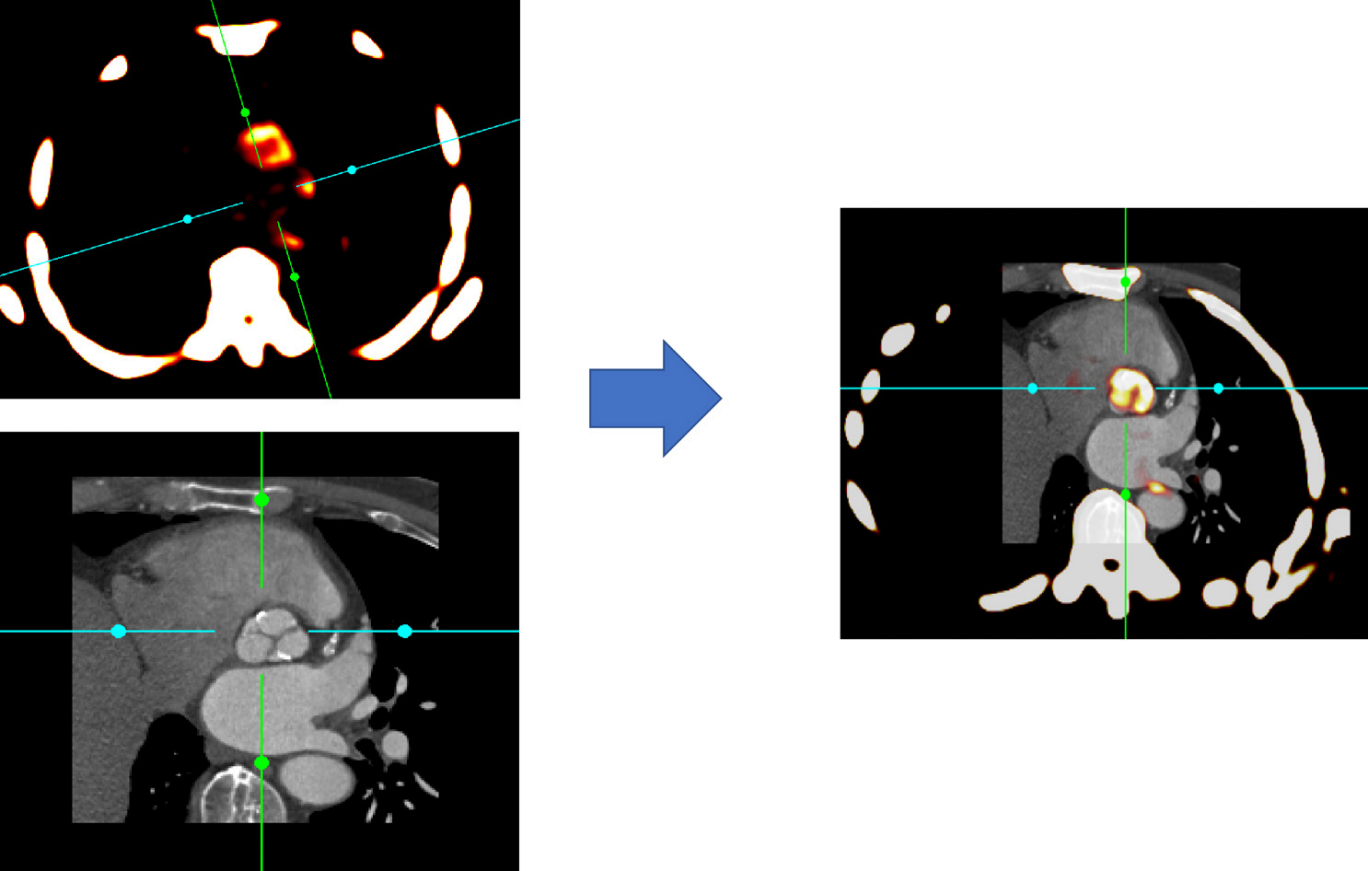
Basis of PET/CT imaging. PET/CT scanners incorporate functional data from PET and anatomical information from CT imaging on the same gantry allowing near simultaneous acquisition. Fused PET/CT images then allow localization of specific pathological processes to individual structures such as the aortic valve in this example. (Images reconstructed using FusionQuant)
